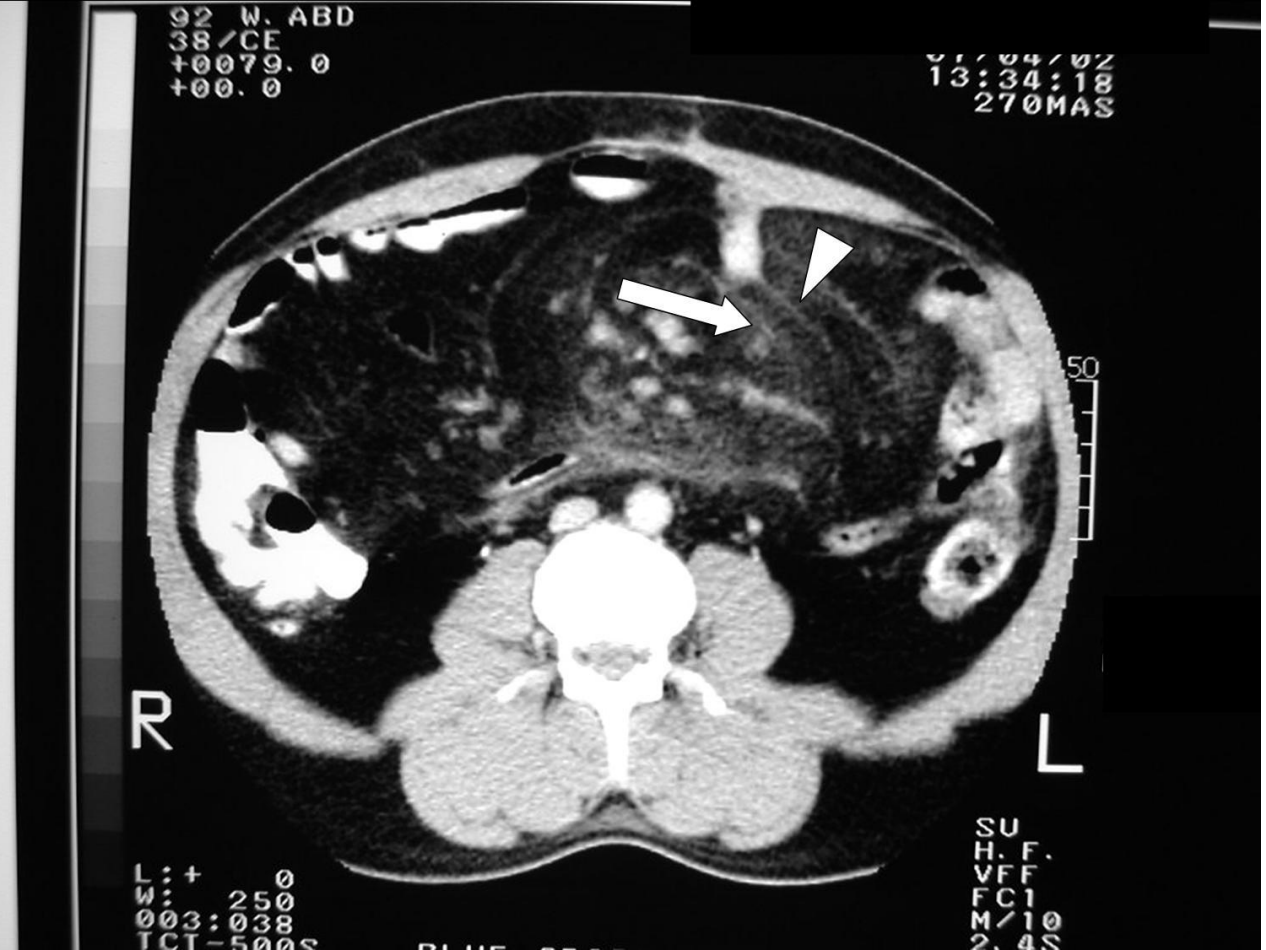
Pre-operative abdominal computed tomography scan of the patient demonstrating characteristic features of mesenteric panniculitis; 'misty mesentry' (*white arrow*) and whorling/stranding of the mesentry (*arrowhead*).
